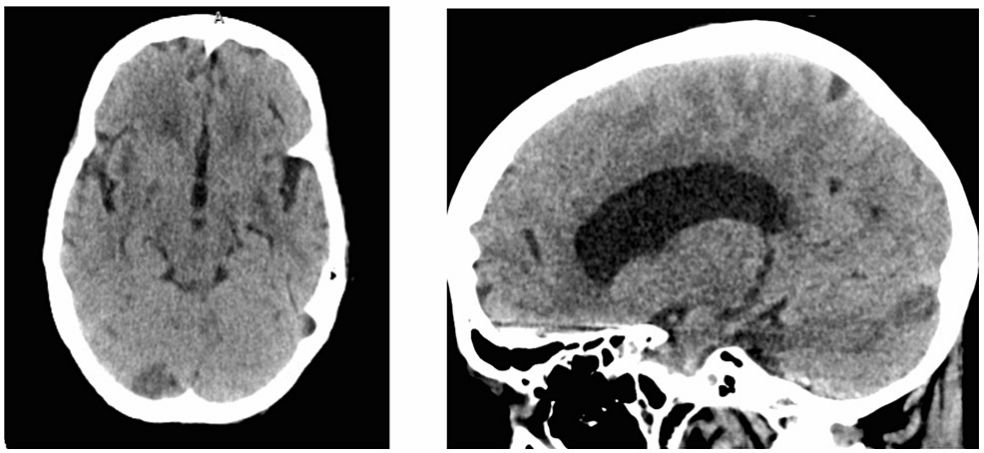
Head CT on February 16, 2022 Impression: Hypodensity in the posterior medial portion of the right occipital lobe, suggesting an acute to subacute infarct.
